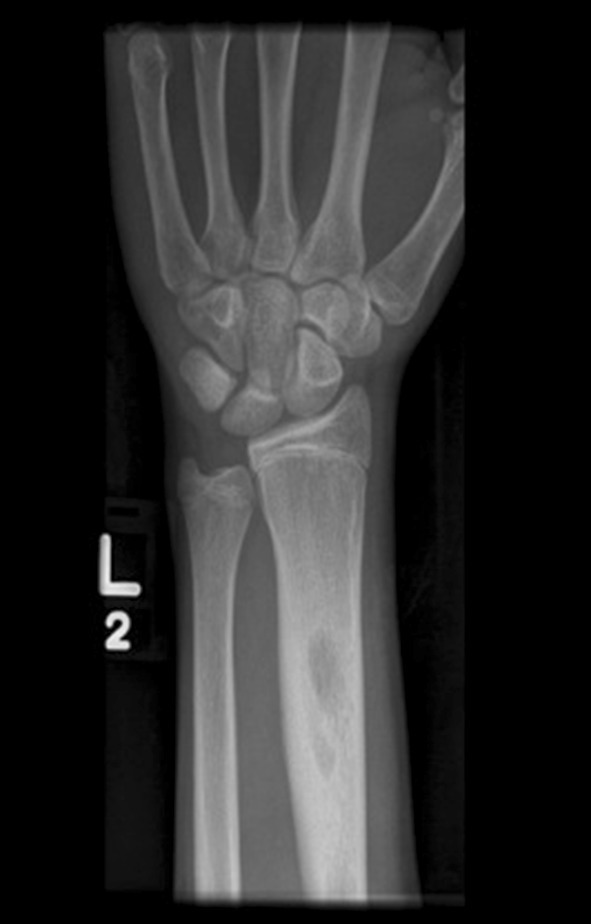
AP radiograph of the *left* distal radius showing an intramedullary lytic lesion
